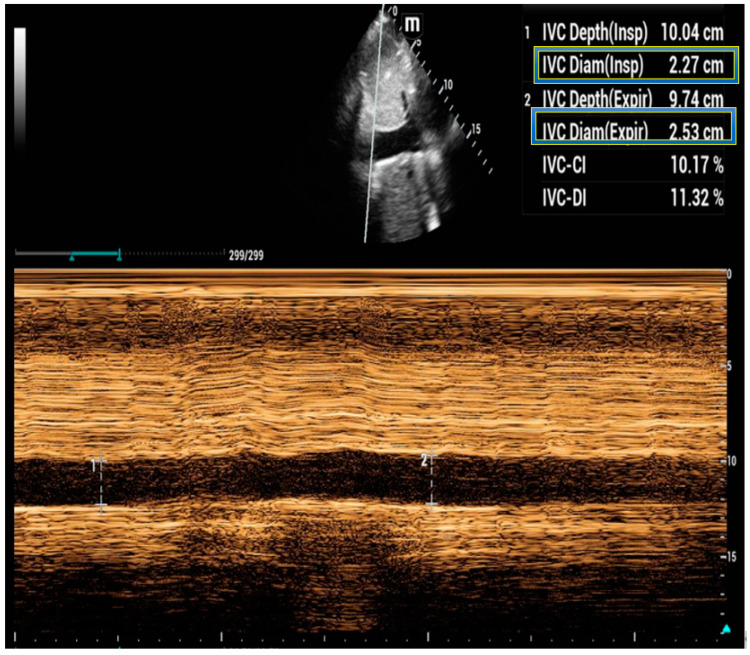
IVC ultrasound showing a diameter >2.1 cm with <50% change during the respiratory cycle.
